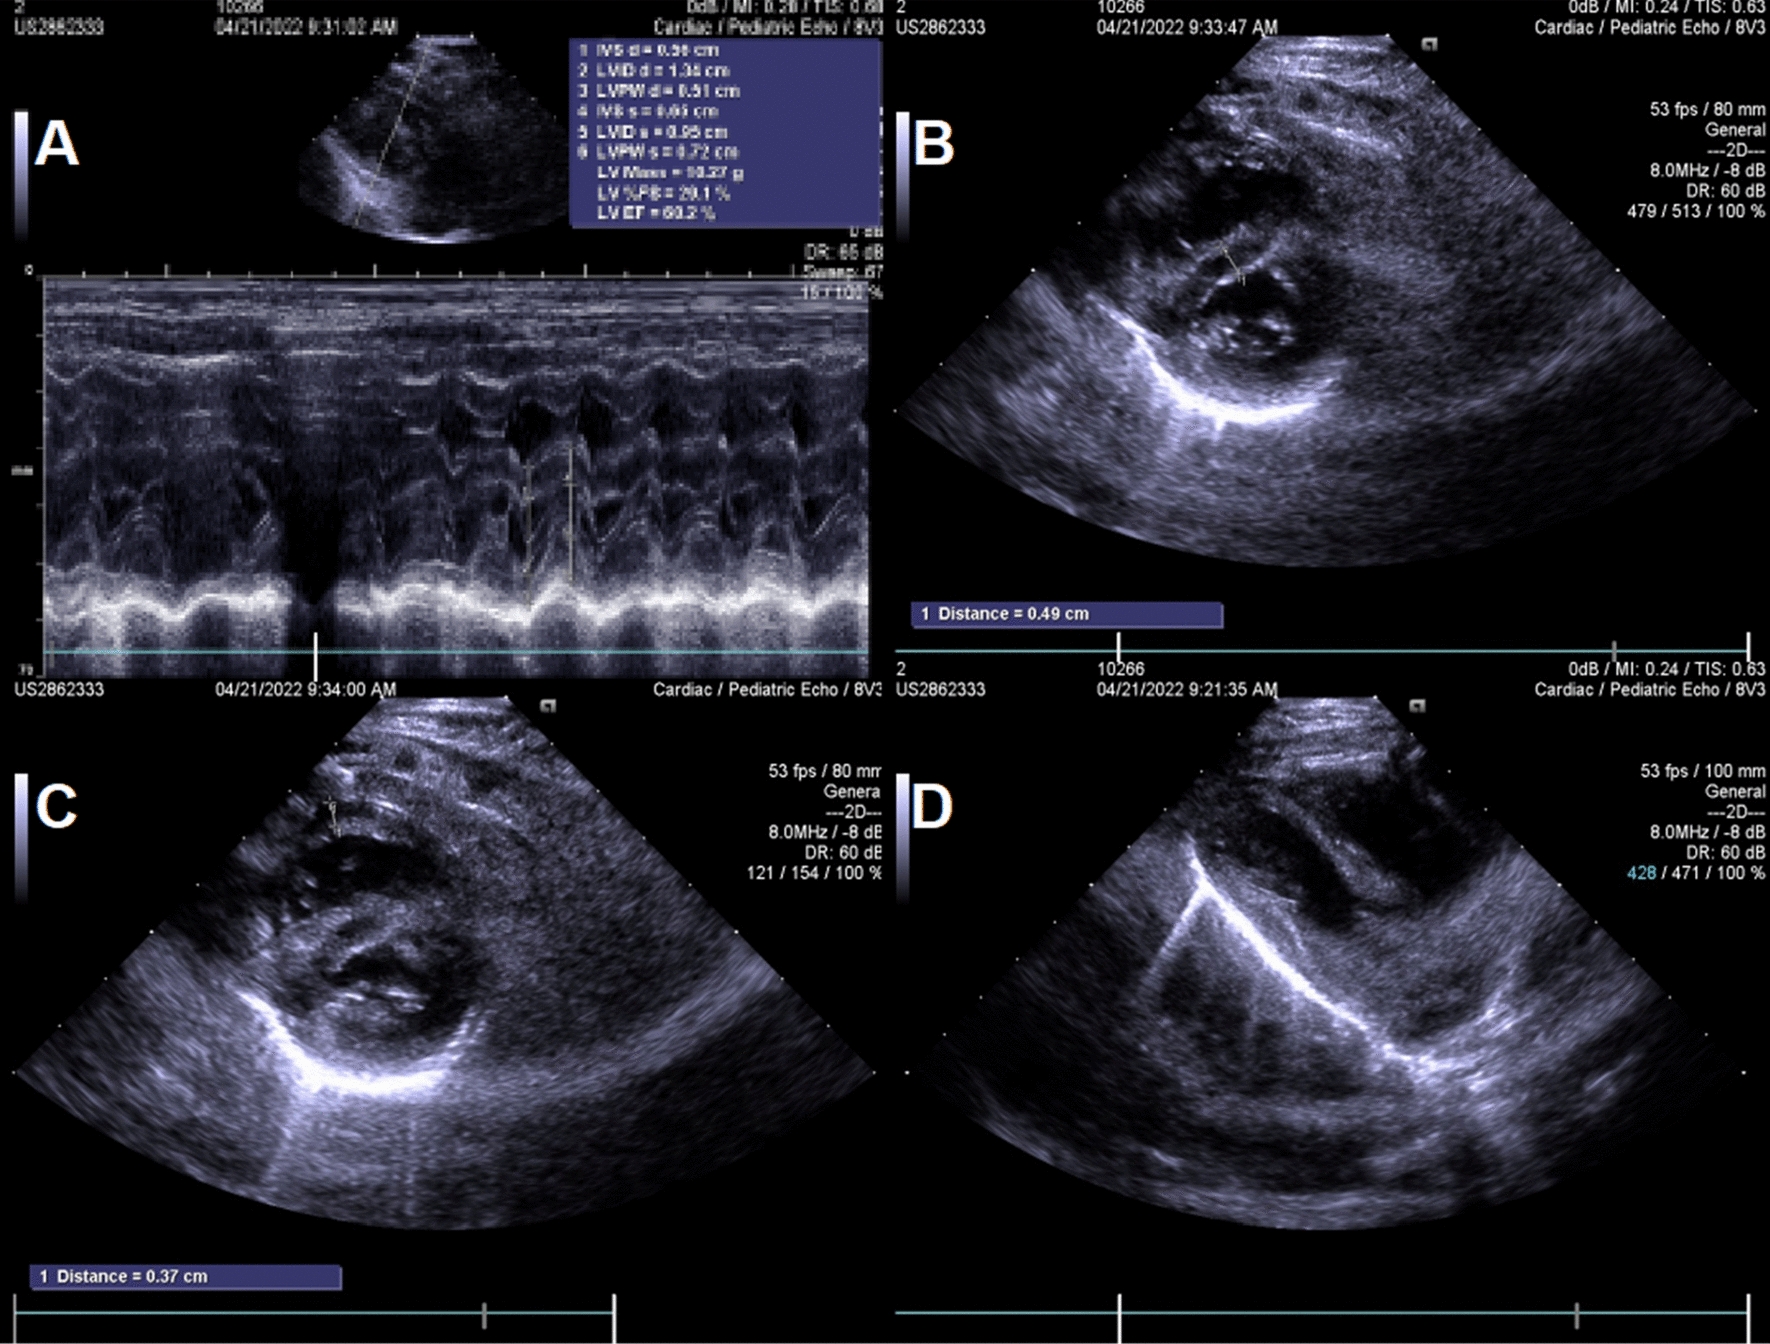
Two-dimensional echocardiogram in follow-up 2 months later. A Cardiac function EF: 60% and FS 29%. B Interventricular septum 5 mm. C Forearm of right ventricle 4 mm. D Stenosis of the left ventricle
